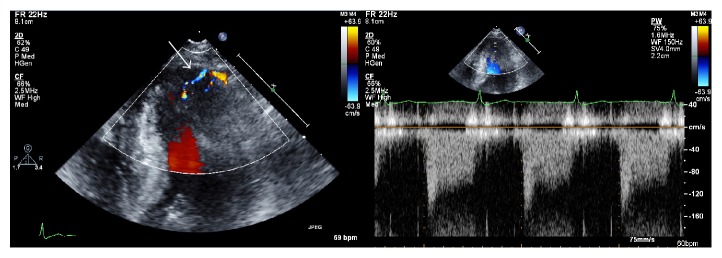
Doppler echocardiography. Color signal visible through the multiple coronary artery fistulas, which directly communicated with the left ventricle, as seen in the apical four-chamber view (left). Pulsed wave Doppler image confirming the diastolic flow of the coronary artery fistulas (right).
